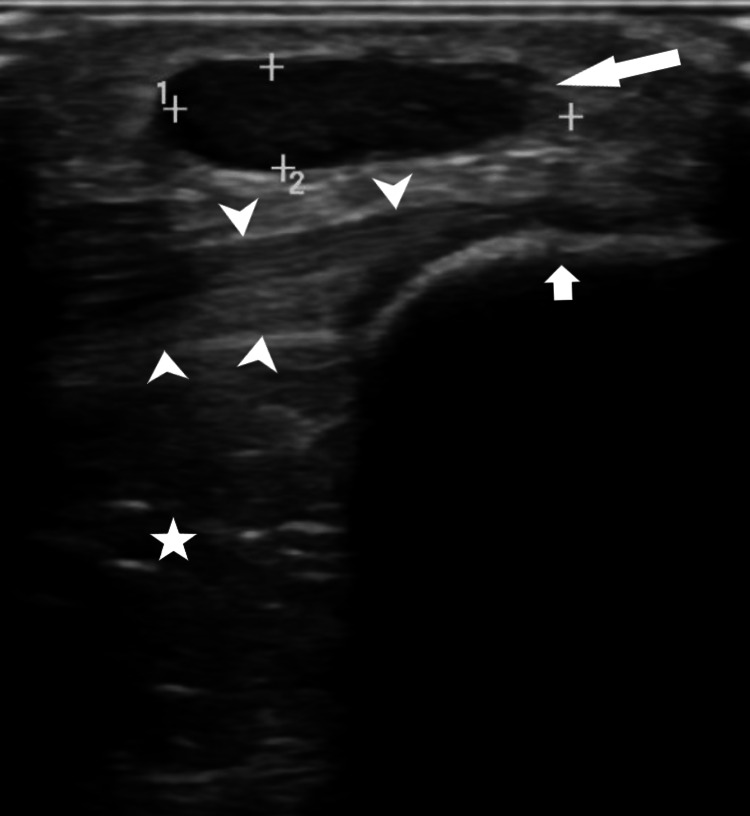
B-mode ultrasonographic examination demonstrates an oval-shaped, well-defined, hypoechoic nodular lesion with mild posterior acoustic enhancement located in the subcutaneous tissue of the prepatellar region, with its long axis parallel to the skin surface (long white arrow). The lesion is noted to be situated immediately anterior to the patella (short white arrow) and the proximal patellar tendon (white triangle arrowheads). No involvement or edema is observed in the infrapatellar fat pad (white star).
